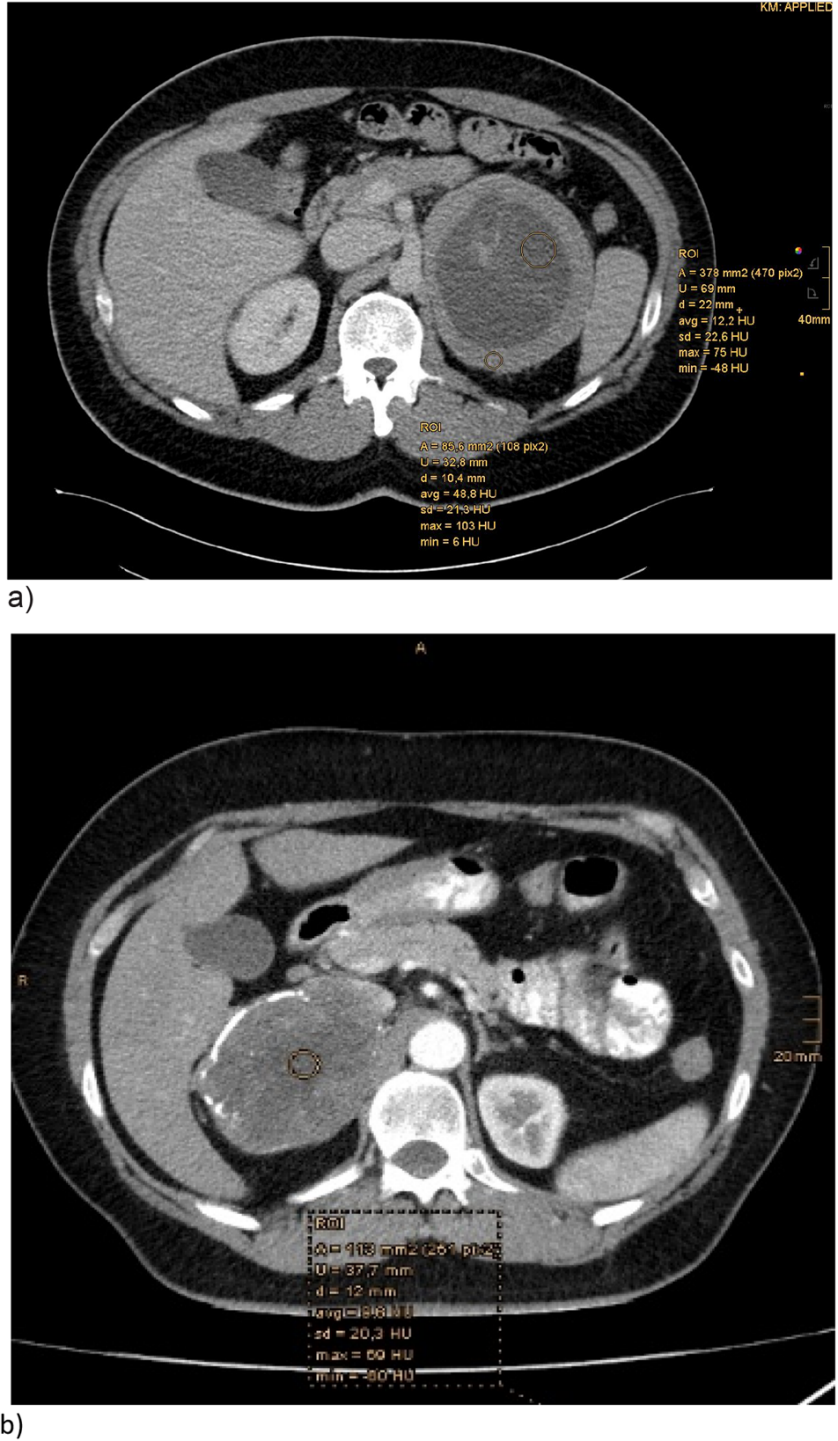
Contrast-enhanced CT scans of both patients. (a) Male patient with a mass of the left adrenal gland. Central parts have 12 HU on average and peripheral areas have about 49 HU in average. Cystic areas, as well as septa, can be seen in this section, central calcifications on further images. (b) Female patient with adrenal tumor of the right adrenal gland displacing the vena cava inferior and an infiltration cannot be barred. Also cystic elements and calcifications are visibly. The tumor has about 9.6 HU on average in the central parts.
